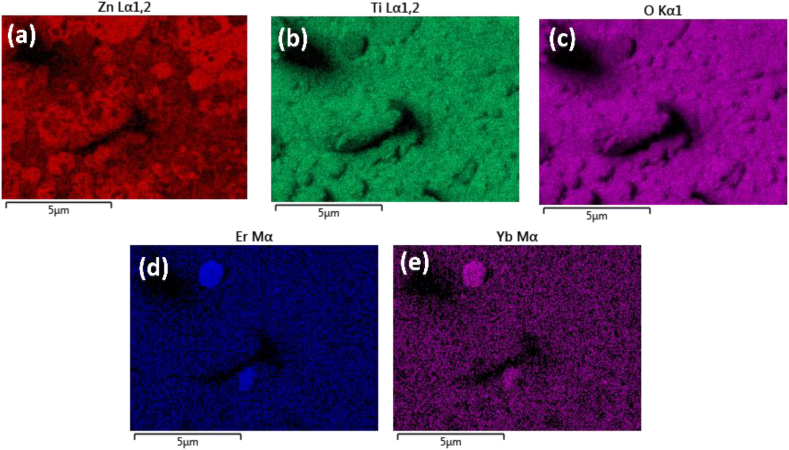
EDS X-ray mapping of the elements in ZnTiO_3_: Er^3+^,Yb^3+^ thin film deposited at 500 °C.
